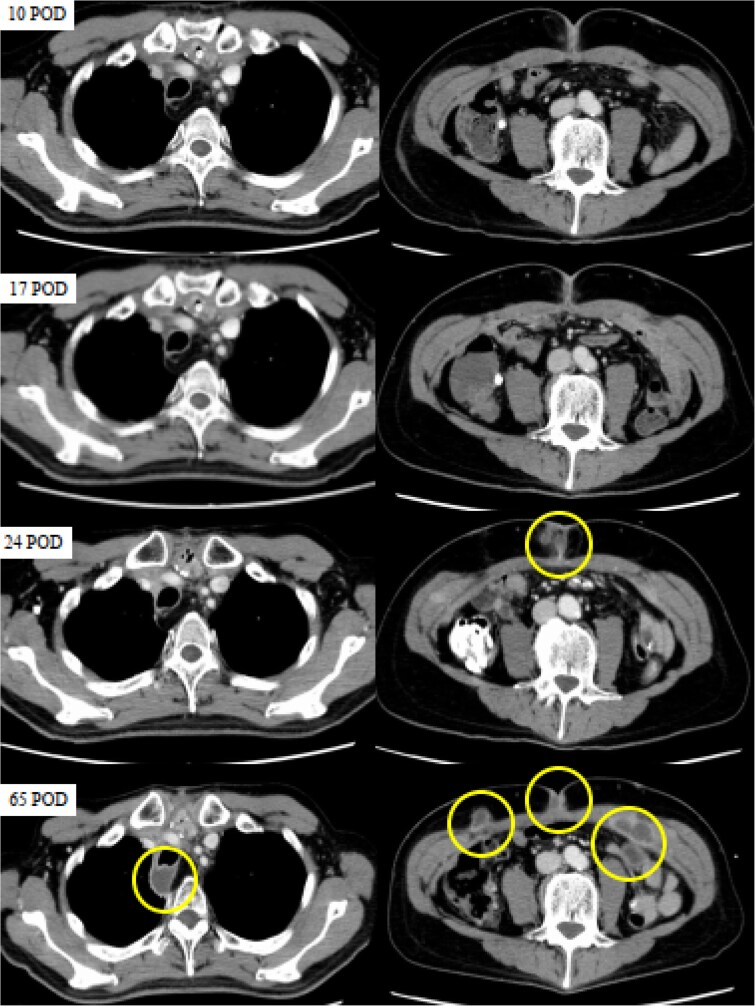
CT images obtained on postoperative days (POD) 10, 17, 24, and 65. The circles indicate abscesses. CT scans were performed on POD 10 and 17 to investigate the high levels of inflammation, but no abnormalities were detected. While no apparent abnormalities were observed on POD 10 and 17, SSI was evident on POD 24, and multiple abscesses had formed in the posterior trachea and abdominal wall by POD 65.
